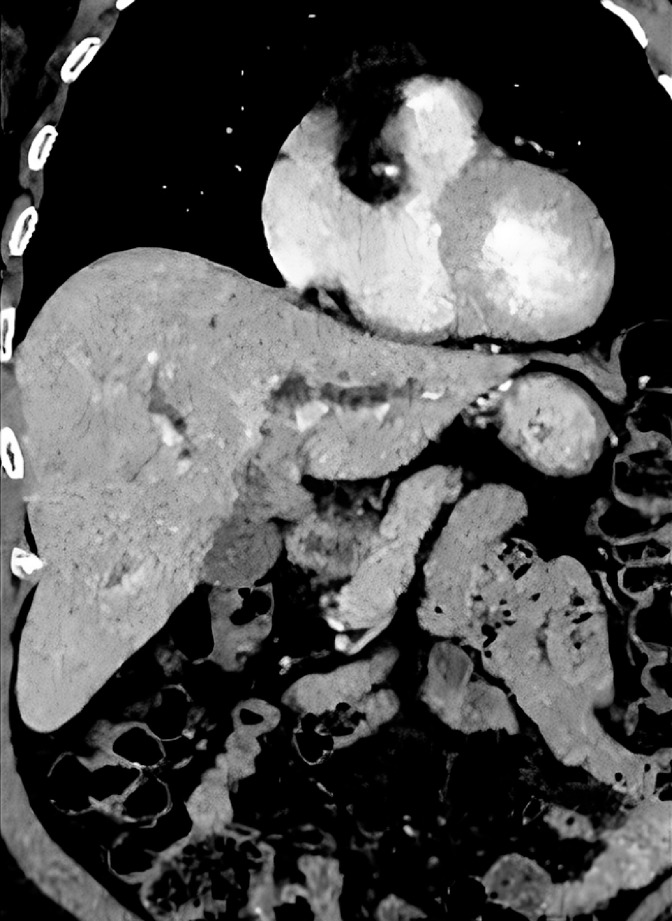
Computed tomography showing circumferential thickening of the proximal common bile duct and left hepatic duct.
